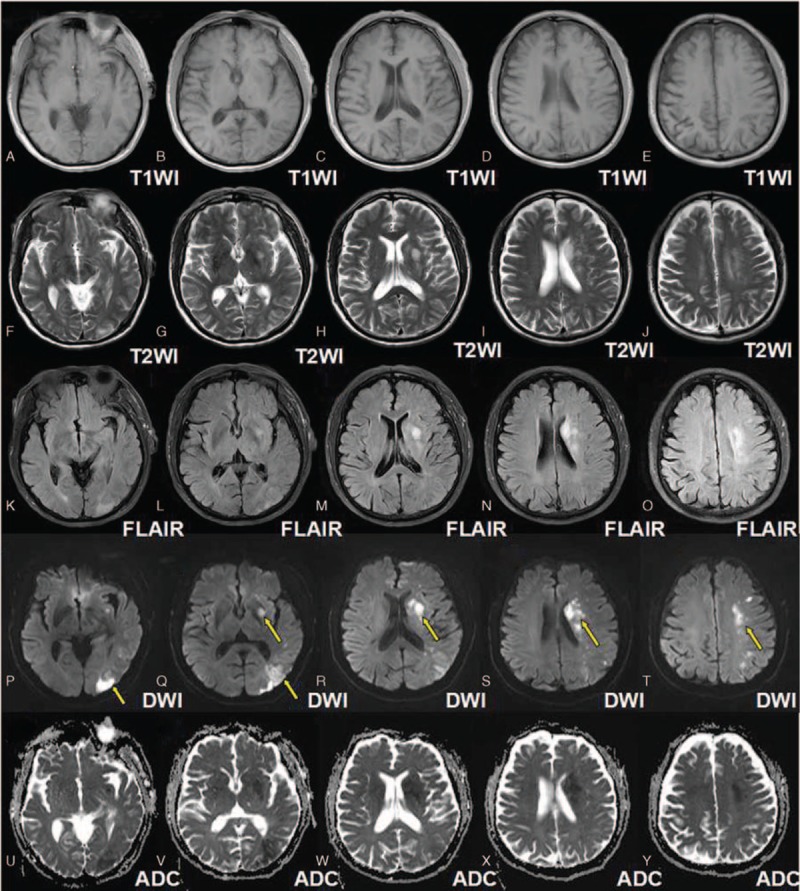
Magnetic resonance imaging panels of the representative case. A–E were T1WI which showed no obvious abnormalities, while E–J and K–O were respectively the T2WI and the FLAIR image which revealed the hyper intense signals predominately involved in the regions of internal capsule, corona radiata, and centrum semiovale. P–T showed more extensive involvements (arrows) on DWI while U–Y the ADC maps consistent with DWI finding. ADC = apparent diffusion coefficient, DWI = diffusion-weighted imaging, FLAIR = fluid attenuated inversion recovery, T1WI = T1-weighted image.
